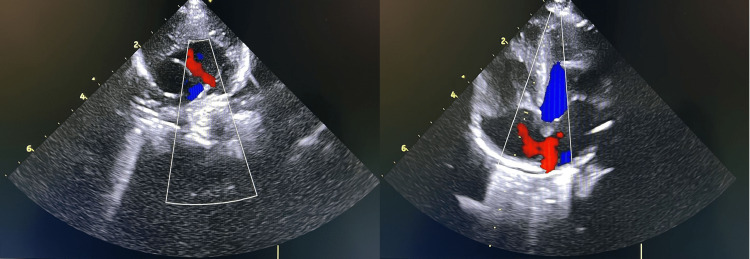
Echocardiogram of case number two Echocardiogram of case number two showing a patent foramen ovale (right) and a small ductus arteriosus (left), both with a left-to-right shunt
